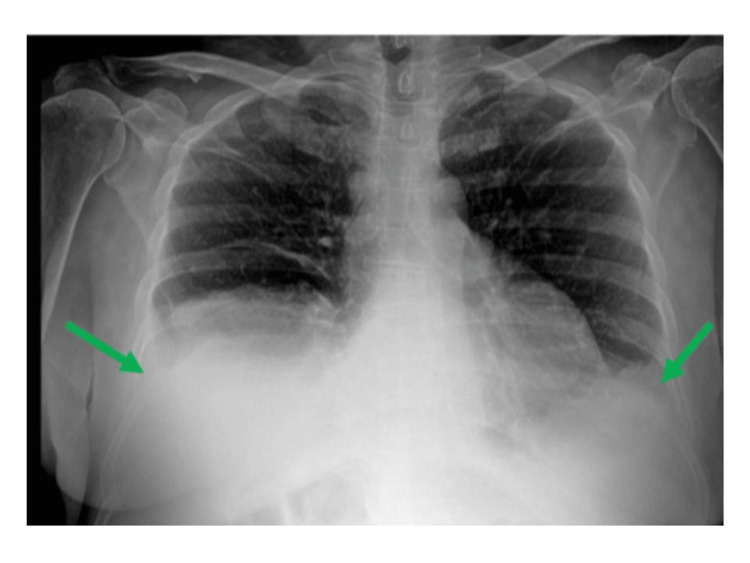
Chest radiograph demonstrating bilateral pleural effusion two months after the initiation of ibrutinib therapy
